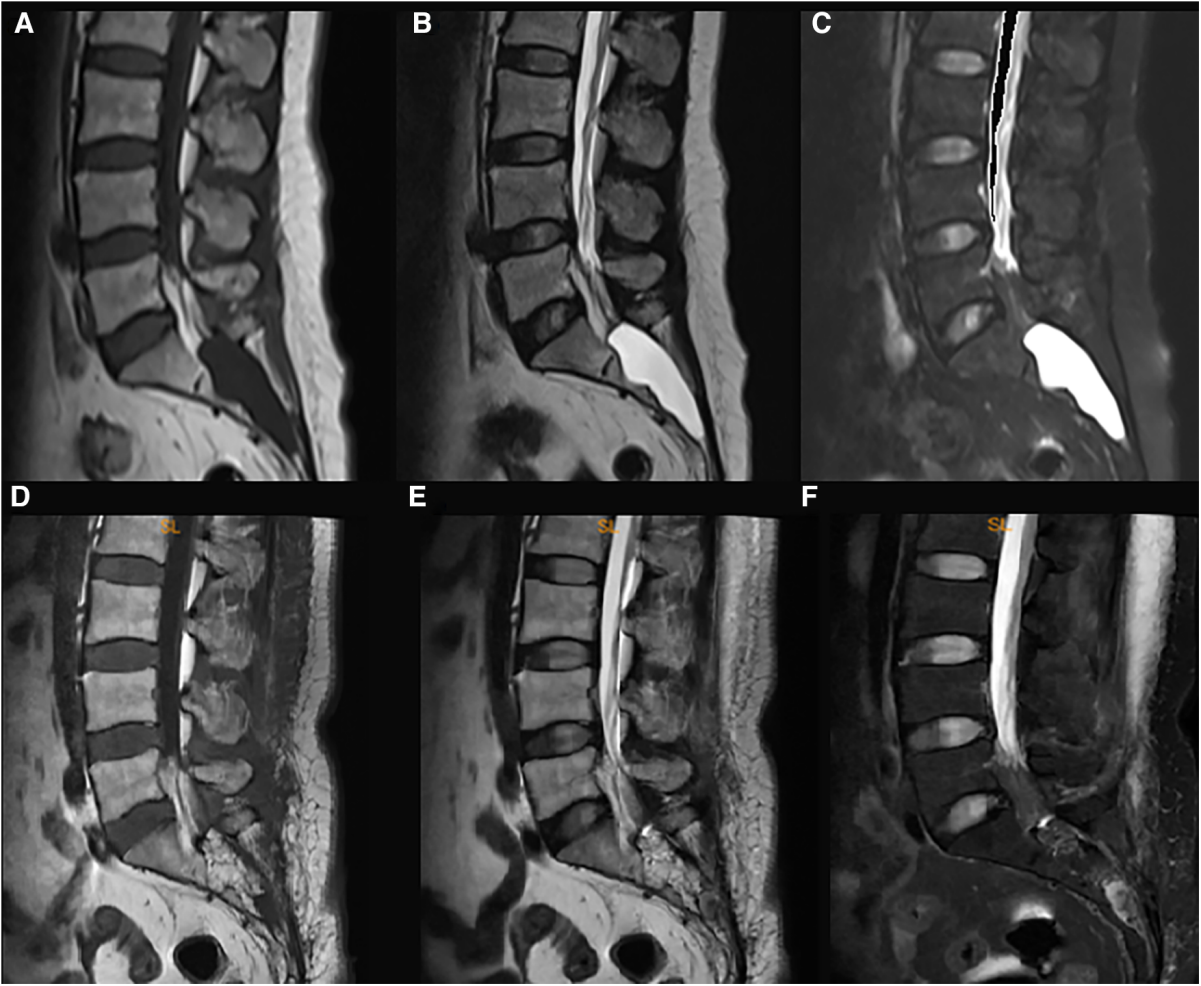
The pre- and post-surgical MR image of a symptomatic Tarlov cyst patient (case 1). Before surgery, the T1-weighted (A), T2-weighted (B), and fat suppression (C) sequences indicated that a Tarlov cyst filled with cerebrospinal fluid was in the sacral canal. After the surgery, the fat tissue occupied the original space of the Tarlov cyst, and no cerebrospinal fluid was detected in the sacral canal anymore (D–F), which indicated no recurrence of the Tarlov cyst.
